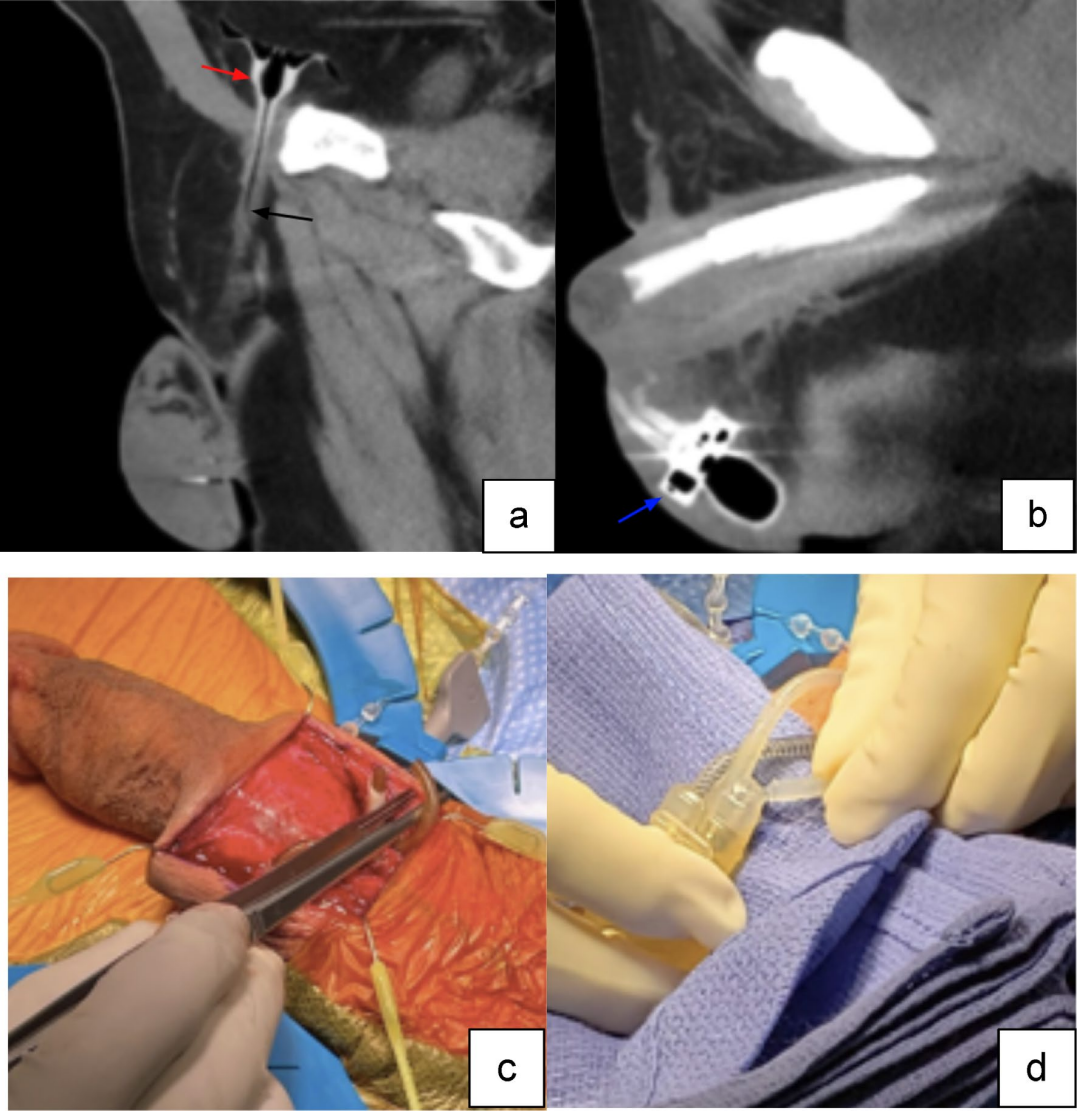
Sagittal (a) and axial (b) CT images without contrast demonstrate a compressed PP reservoir containing air (red arrow), fractured free-floating tubing (black arrow), and air within the scrotal pump (blue arrow). Intraoperative photos of penoscrotal incision at time of repair demonstrate multiple fractures of prosthetic connection tubing (c, d)
